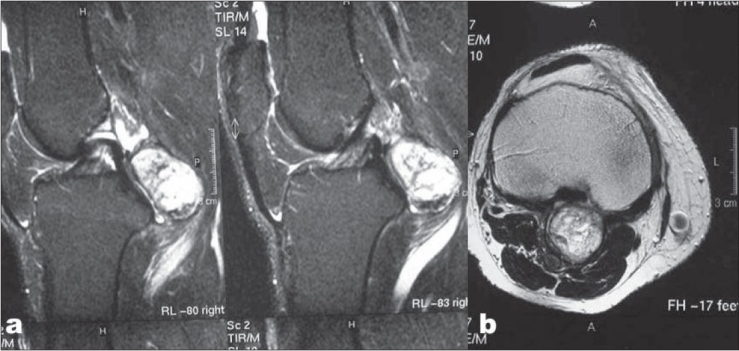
(a) Saggital and (b) axial MR images T2 W showing a hyperintense lesion in the popliteal fossa
